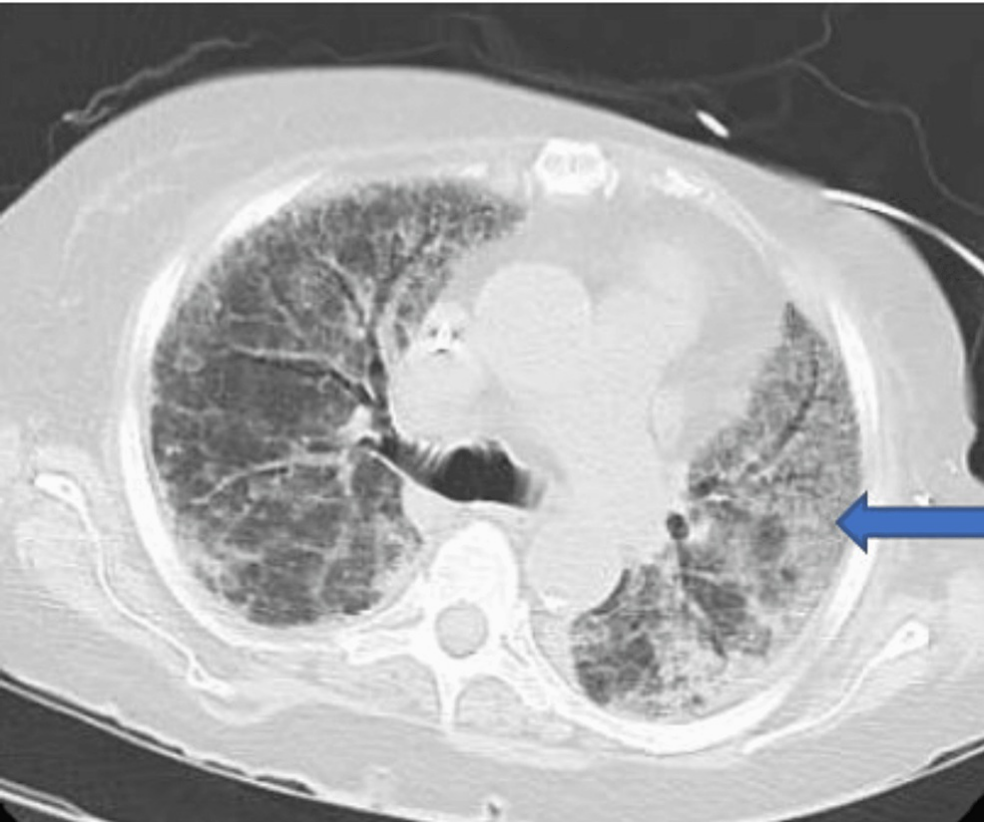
CT chest: blue arrow showing extensive ground-glass infiltrates, extensive fibrotic changes, consolidations at the lung bases, left greater than right.
